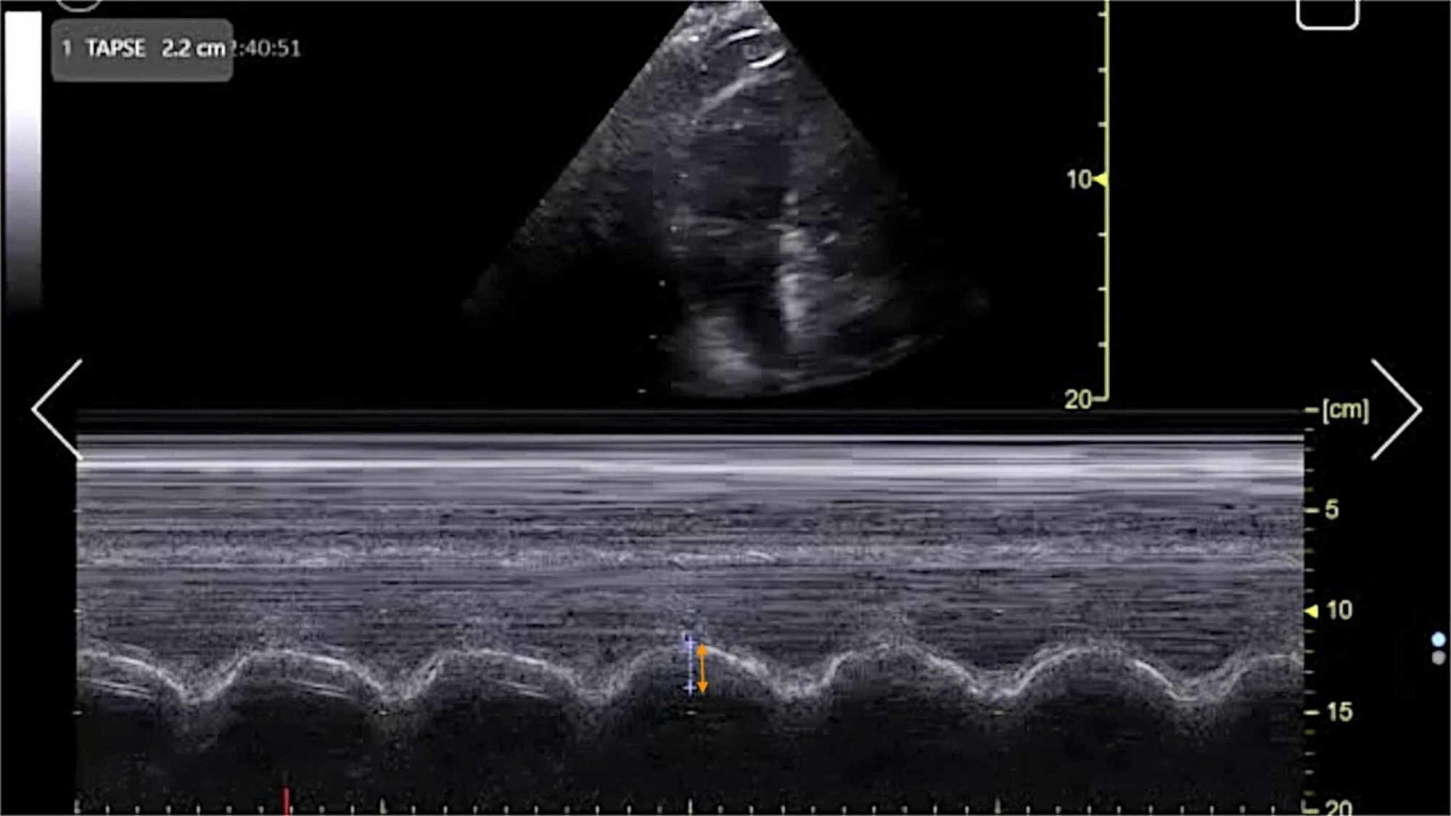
Point-of-care echocardiography 24 hours post-thrombolysis. Apical four-chamber view with M-mode tracing demonstrating TAPSE (orange arrow). TAPSE, tricuspid annular plane systolic excursion.
